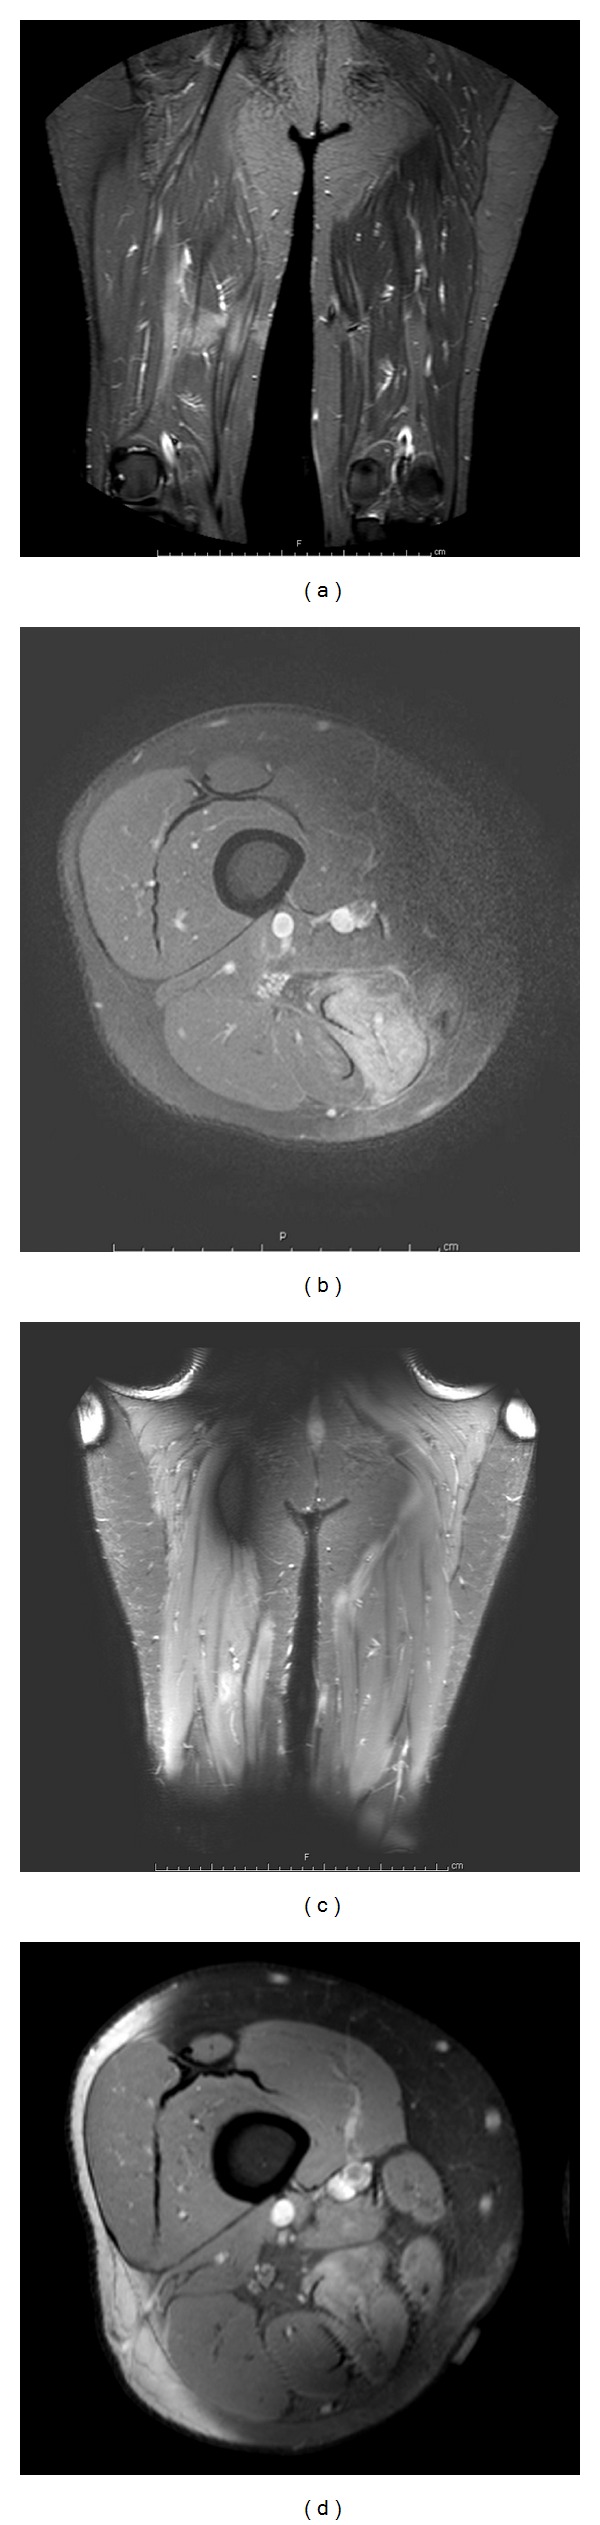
(a) and (b) Initial MRI of the lower extremities demonstrates edema in the right semimembranosis and semitendinosis muscles with abnormal enhancement in the distal sciatic and common peroneal nerves. (c) and (d) Delayed MRI of the lower extremities demonstrates near resolution of edema in the muscle and normalization of the nerves without abnormal enhancement.
